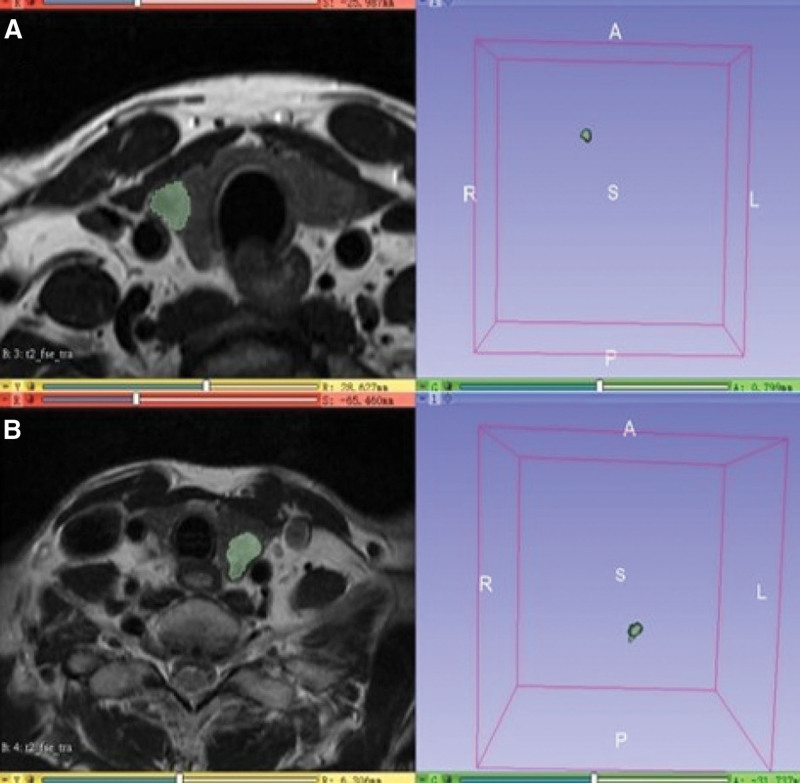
ROI segmentation based on transverse T2WI. (A) Image from a representative PTC patient without ETE (female, 47 years old). (B) Image from a representative patient with ETE (female, 37 years old). ETE = extrathyroidal extension, PTC = papillary thyroid carcinoma, ROC = receiver operating characteristic, T2WI = T2-weighted imaging.
